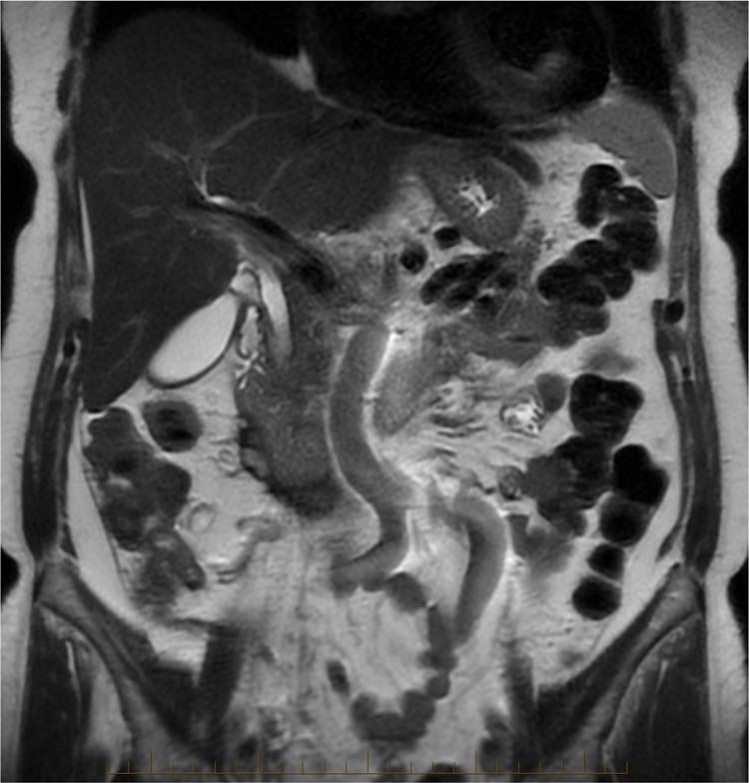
MRI abdomen, coronal view. Resolving paraduodenal hematoma.
